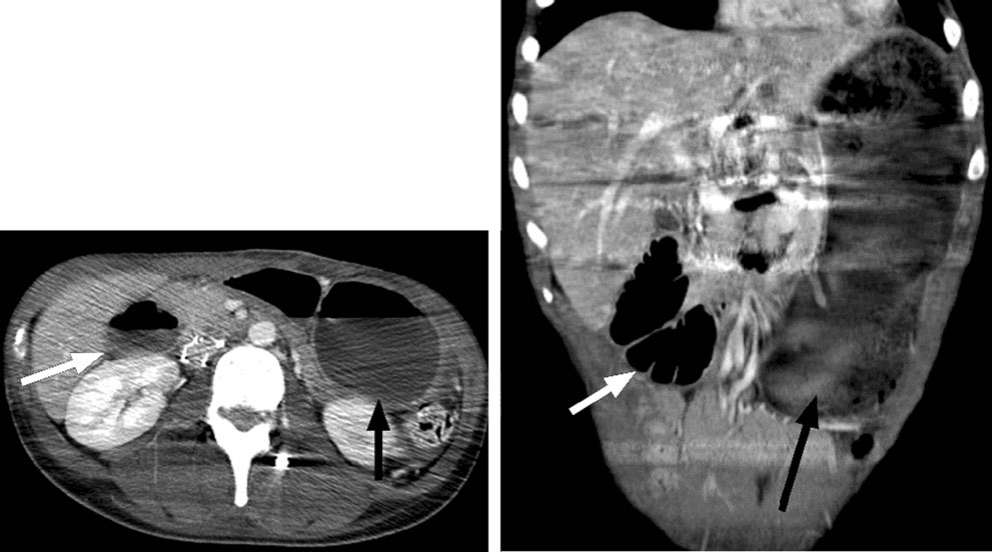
SMA syndrome. Axial and coronal CT images show the distended, fluid-filled stomach (*black arrows*) and mild dilation of the proximal duodenum (*white arrows*). The duodenum is narrowed as it crosses the spine under the superior mesenteric artery and the remaining small bowel is small in caliber (not shown). Note the substantial streak artifact from the spinal fusion hardware
